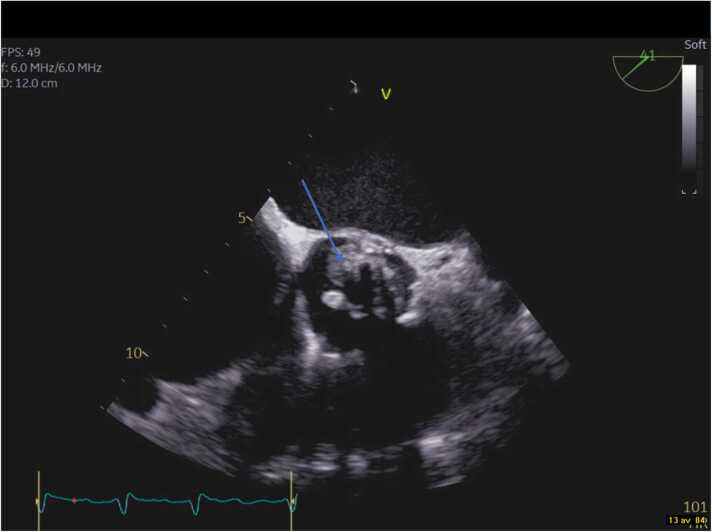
Image from transoesophageal echocardiography at mid-esophageal position. The aortic valve bioprosthesis is visualised in short-axis view, the arrow indicates the vegetation.
